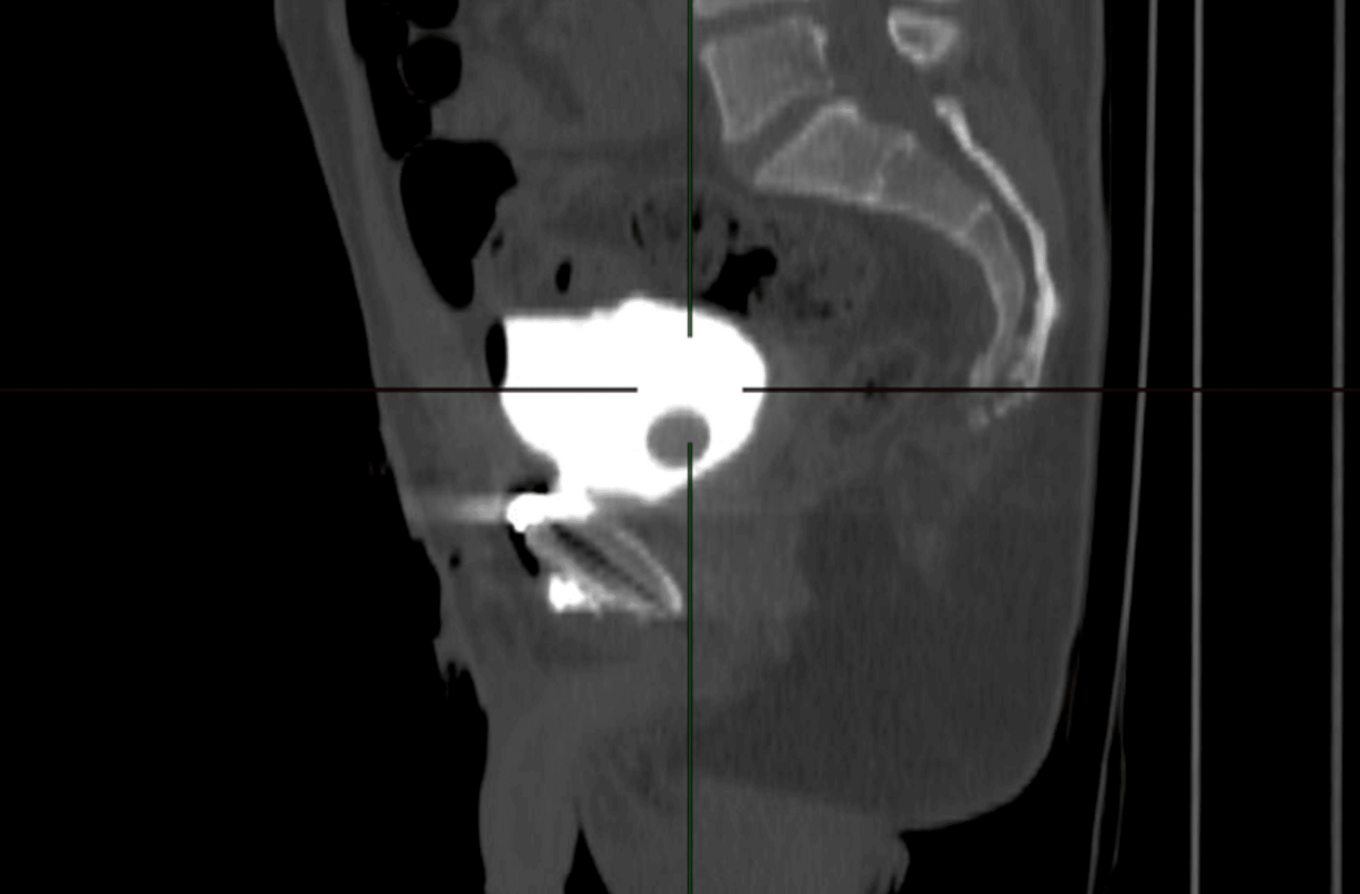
Retrograde CT cystography showing resolution of the VCF with no contrast extravasation
